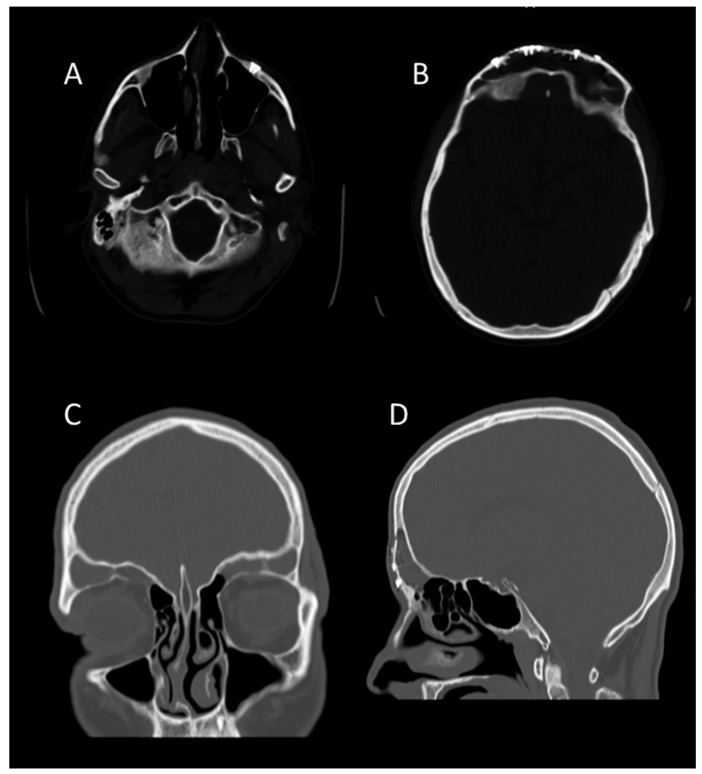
CT-scan aspect months after surgery. (A) the bone consolidation at the level of the lower left orbital rim and the symmetry of the bone contours at this level are highlighted; (B) bone consolidation with anatomical restoration of the anterior walls of the frontal sinuses; (C) coronal section that highlights the symmetry of the orbital dimensions, the symmetry of the frontal and ethmoidal sinuses contours; (D) sagittal section that highlights the anatomical position bone consolidation at the glabellar and nasal level.
